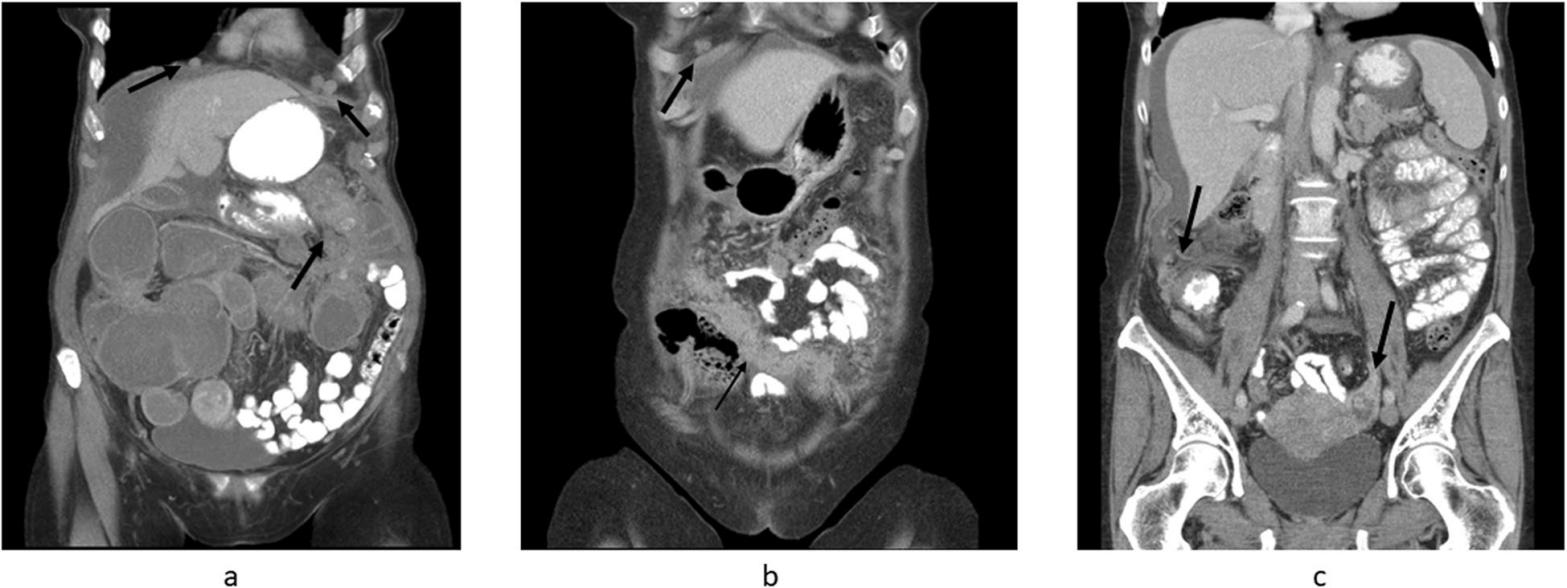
Peritoneal carcinomatosis and CPLN in women with ovarian cancer. a CT-image (coronary projection) showing bilateral enlarged CPLN and peritoneal carcinomatosis primarily affecting left side intestines, causing obstruction. b CT-image (coronary projection) showing ventral carcinomatosis, i.e. omental cake and enlarged CPLN. c CT-image (coronary projection, same examination as b) showing a left sided ovarian mass and diffusely spread carcinomatosis primarily affecting right sided colon
